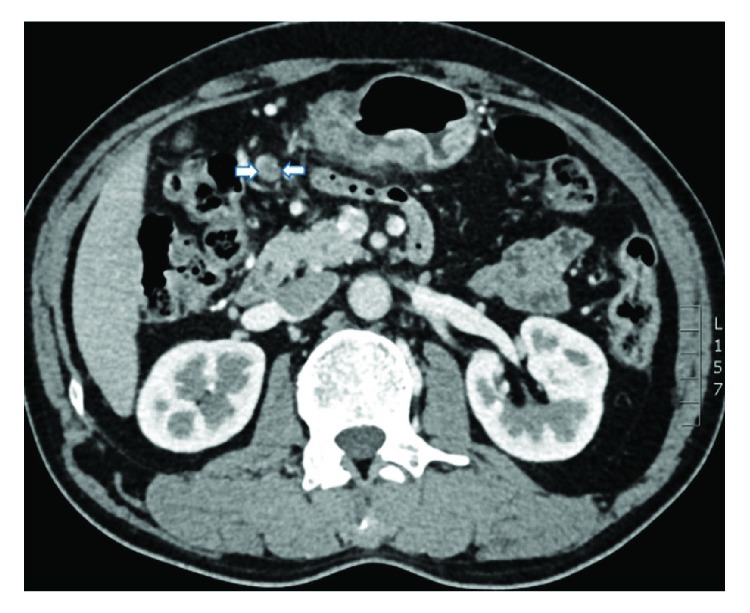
CT image obtained in a 54-year-old man. A round-shaped lymph node with a central low-attenuation area is visualized beside the right gastric artery.
